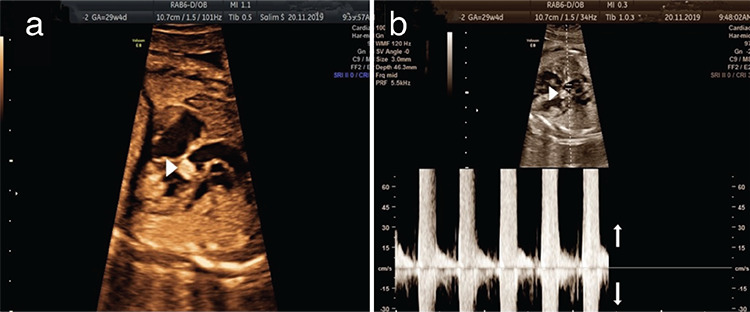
A rhabdomyoma is located at the level of the aortic valve (arrow head) (a). Power Doppler obtained just above the tumor at the beginning of the ascending aorta (b). Bidirectional flow demonstrated aortic regurgitation (arrows)
